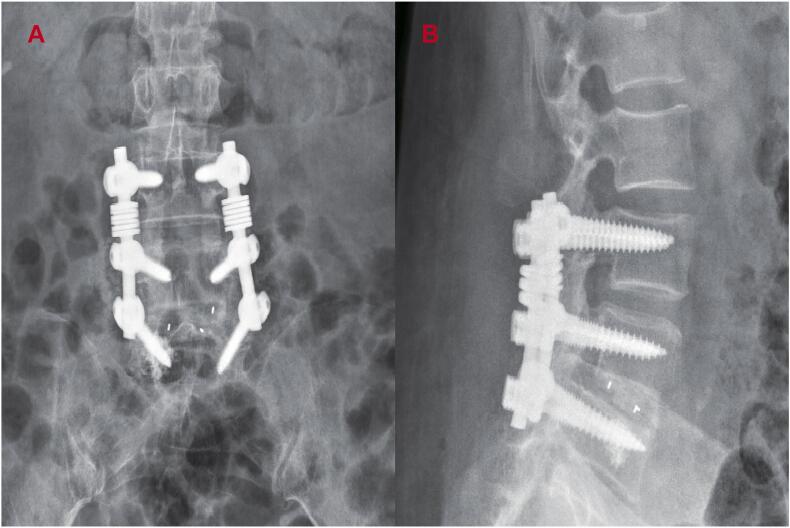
A-B. Anteroposterior (A) and lateral (B) radiographs after the spinal revision surgery showed correct positioning of the pedicle screws.
